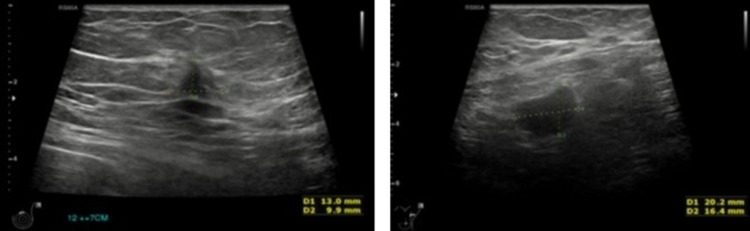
Left breast ultrasound within the left breast at the 12 o'clock position plus 7 cm from the nipple, there is an ill-defined hypoechoic lesion of 13 x 10 x 10 mm (volume 0.7 ml).
